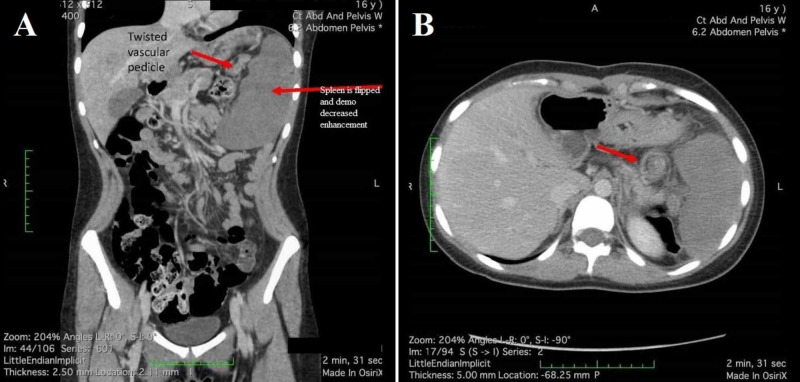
CT with contrast CT with contrast demonstrating splenic infarct secondary to torsion in a 16-year-old female patient. (A) Sagittal view with noted splenic enlargement and twisted vesicular pedicle. (B) Axial view of splenic infarction.
